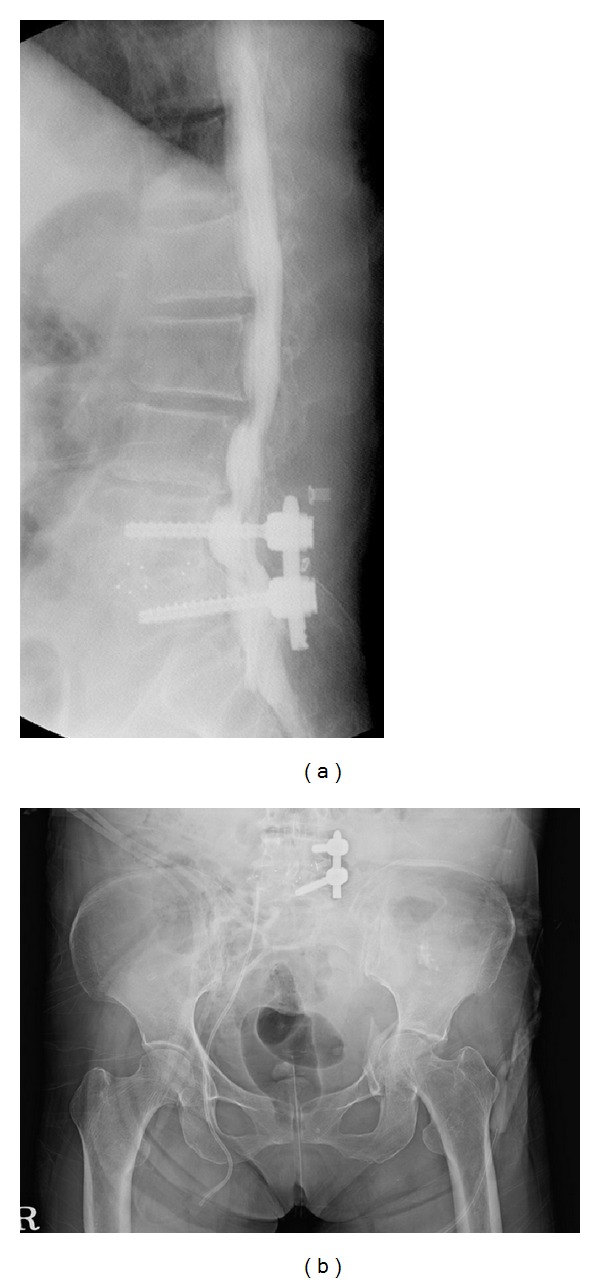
(a) Myelography taken after emergency care showing instrument on L4-5. (b) Radiograph taken 2 days after seizure episode showing left acetabular fracture with medial displacement of femoral head.
